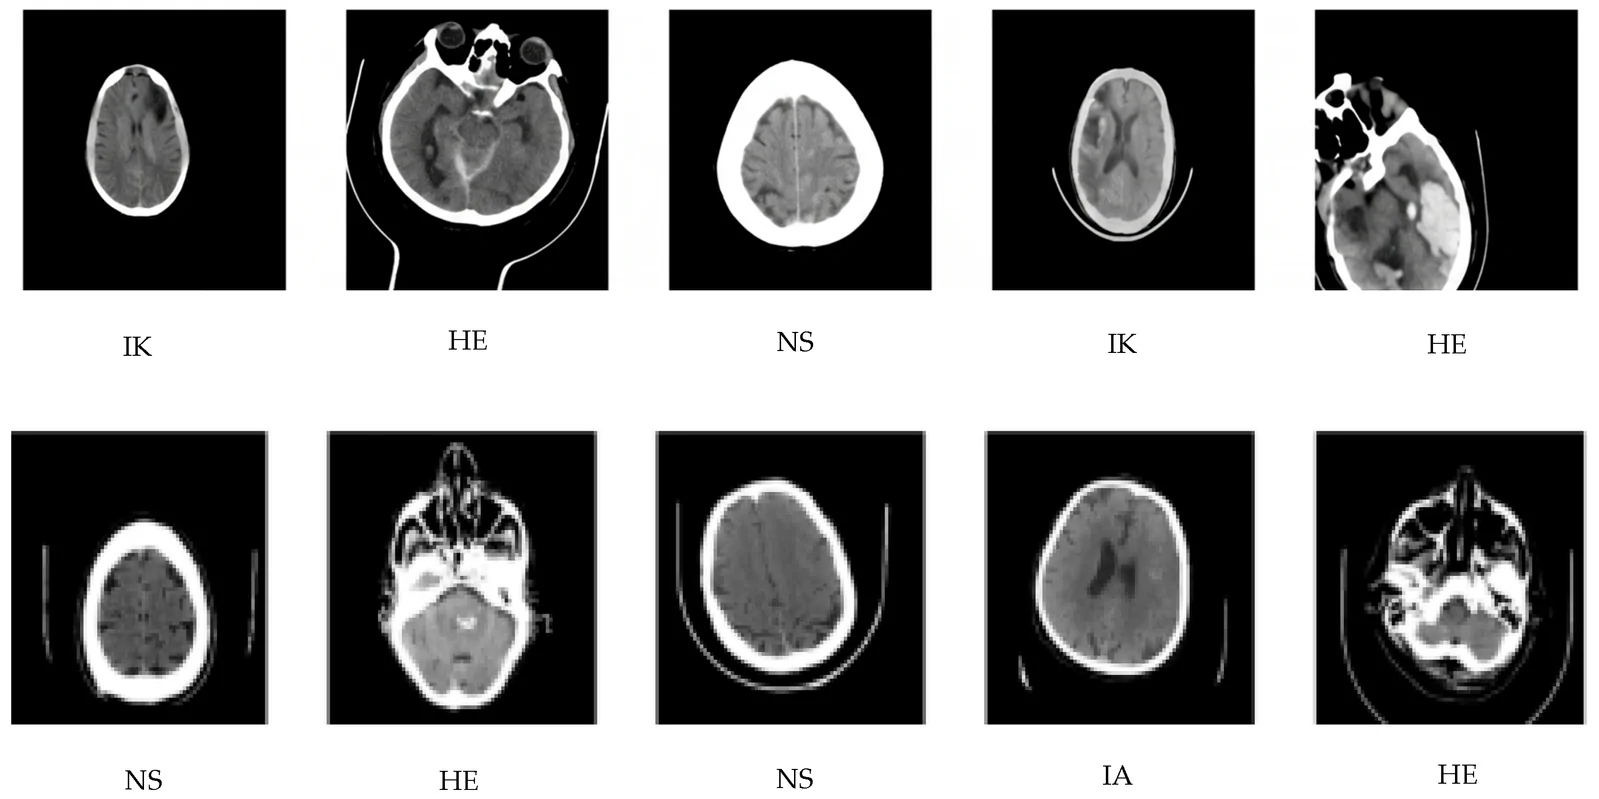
Representative cranial CT images from StrokeCT-2C5K.
